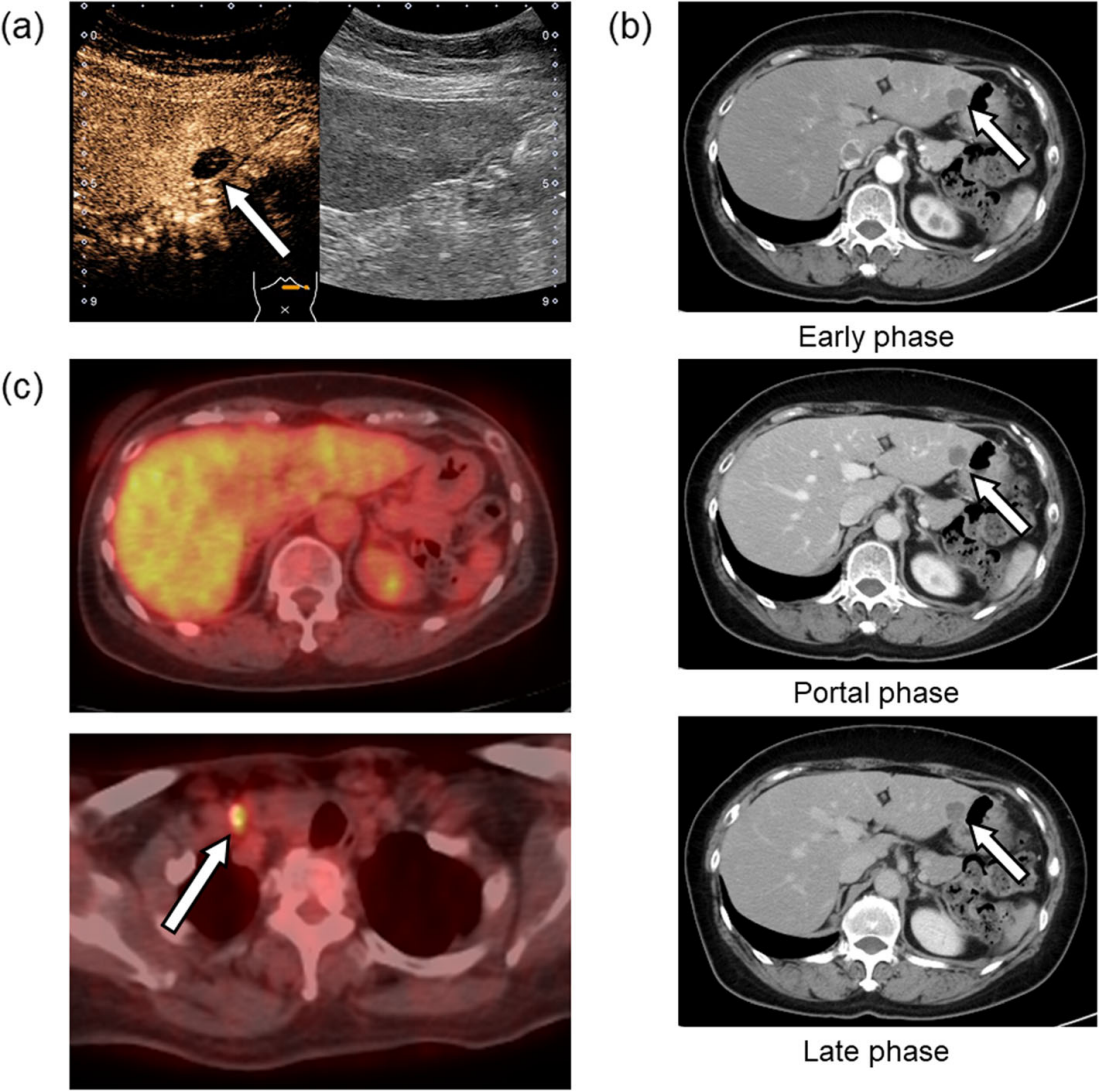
a Abdominal contrast-enhanced ultrasonography shows a 16-mm echo-free mass in the lateral segment of the liver, which resulted in a defect in the Kupffer phase (arrow). b Enhanced abdominal computed tomography scan shows a 16-mm marginally enhanced tumor in the lateral segment of the liver (arrow). c Fluorodeoxyglucose positron emission tomography (FDG-PET) is negative for liver tumors. FDG uptake (maximal standardized uptake value = 3.43) is observed at the nodule outside the right lobe of the thyroid gland (arrow)
